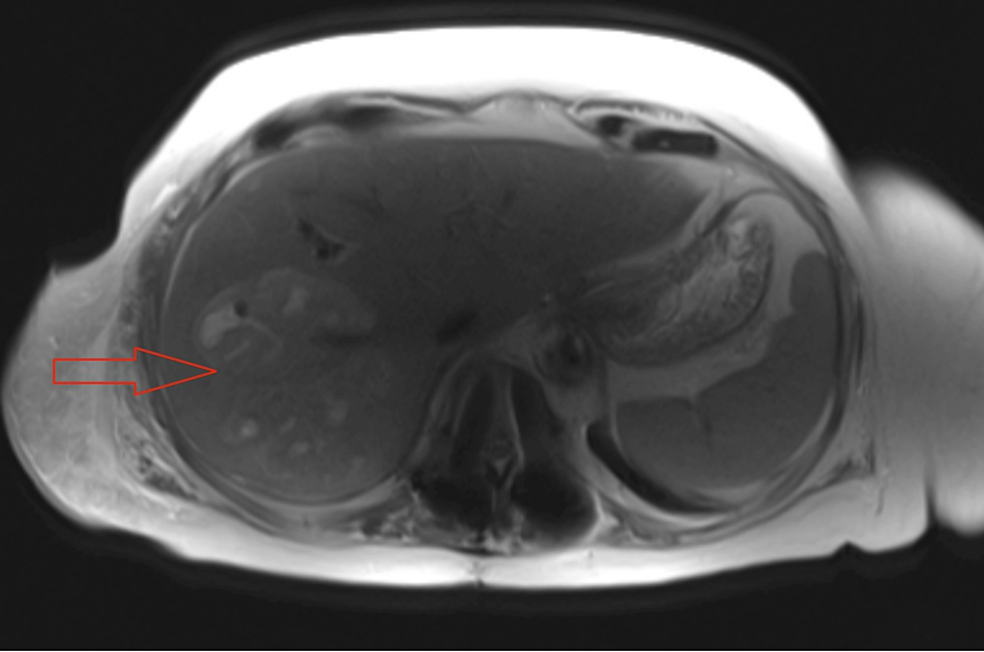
Multiple liver lesions are present on the MRI of the abdomen with contrast axial section.
